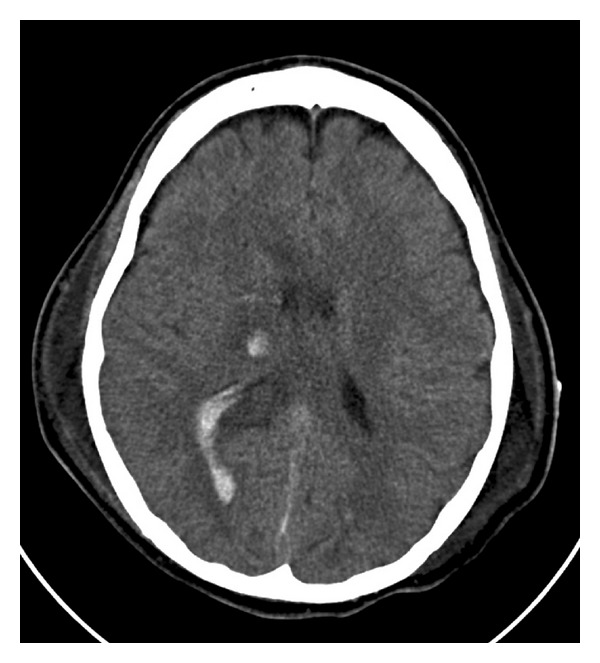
CT of head demonstrates intraventricular and intraparenchymal blood.
